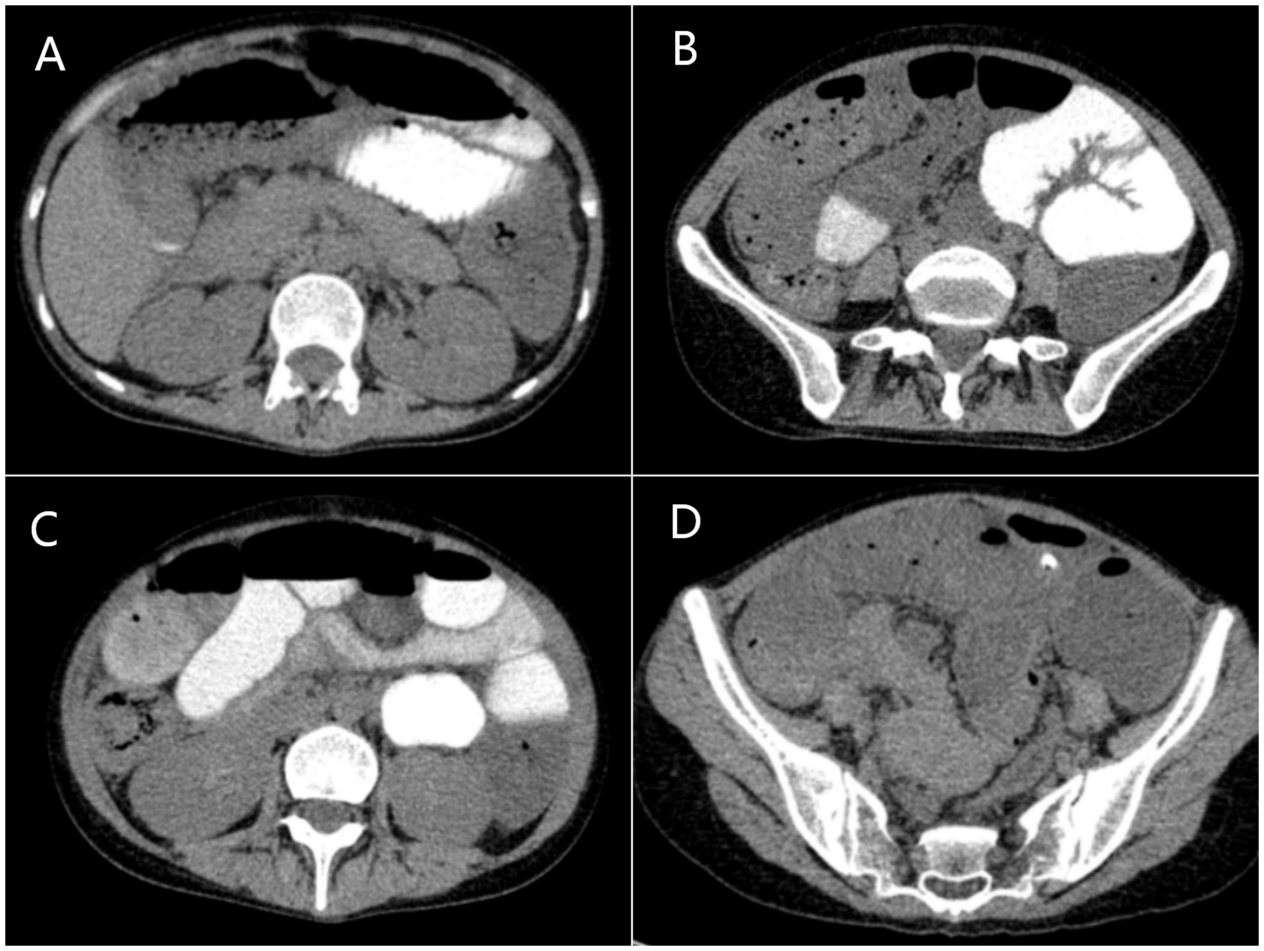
The abdominal CT during the second episode of bowel obstruction. (A–D) Axial scans showing gas accumulation and bowel dilation in the small intestine.
